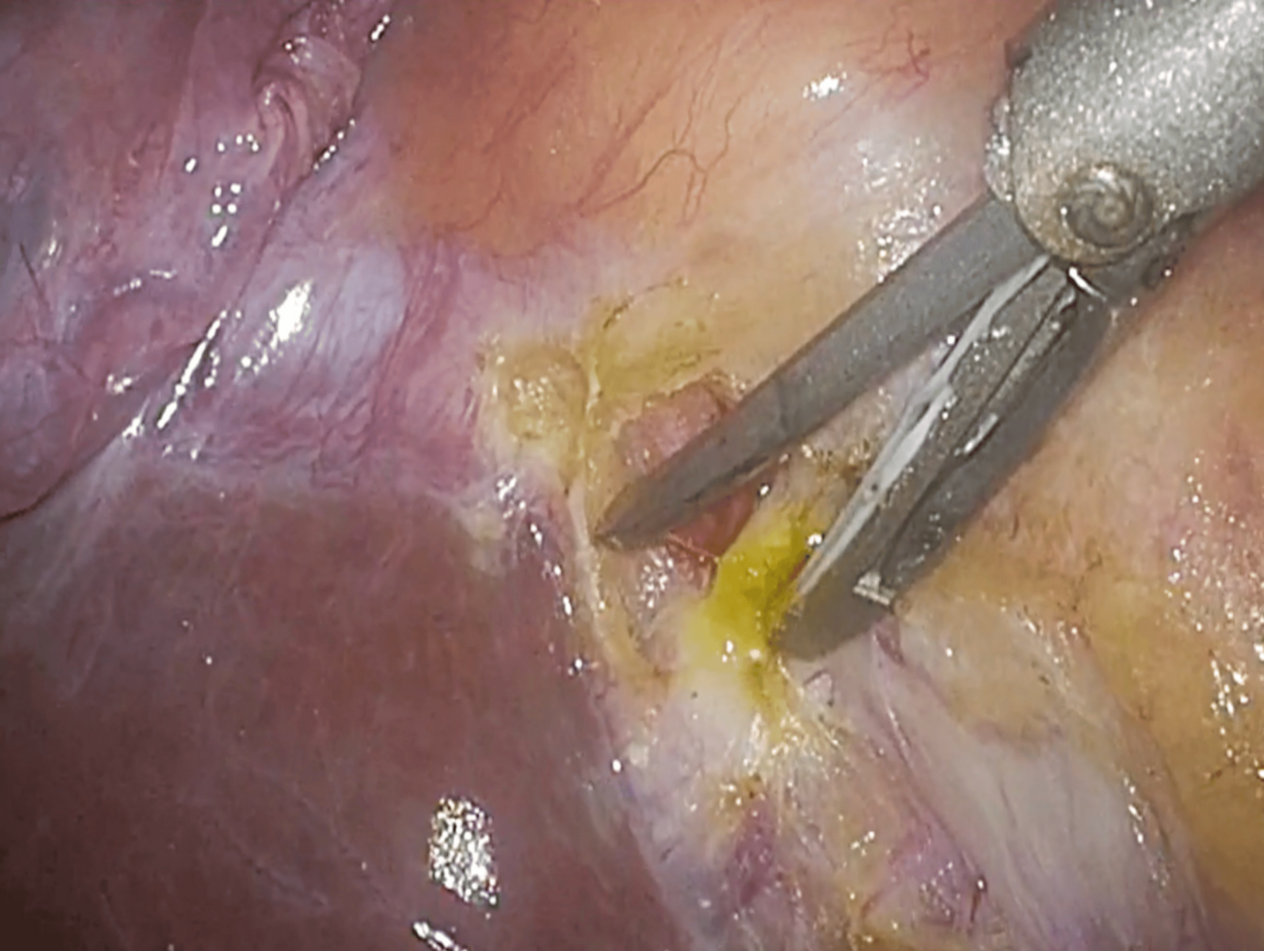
Bile Leakage During dissection of the dorsal side of the gallbladder, a bile duct was inadvertently transected when the blade of the ultrasonic coagulation and cutting device came into contact with it, causing bile leakage.
